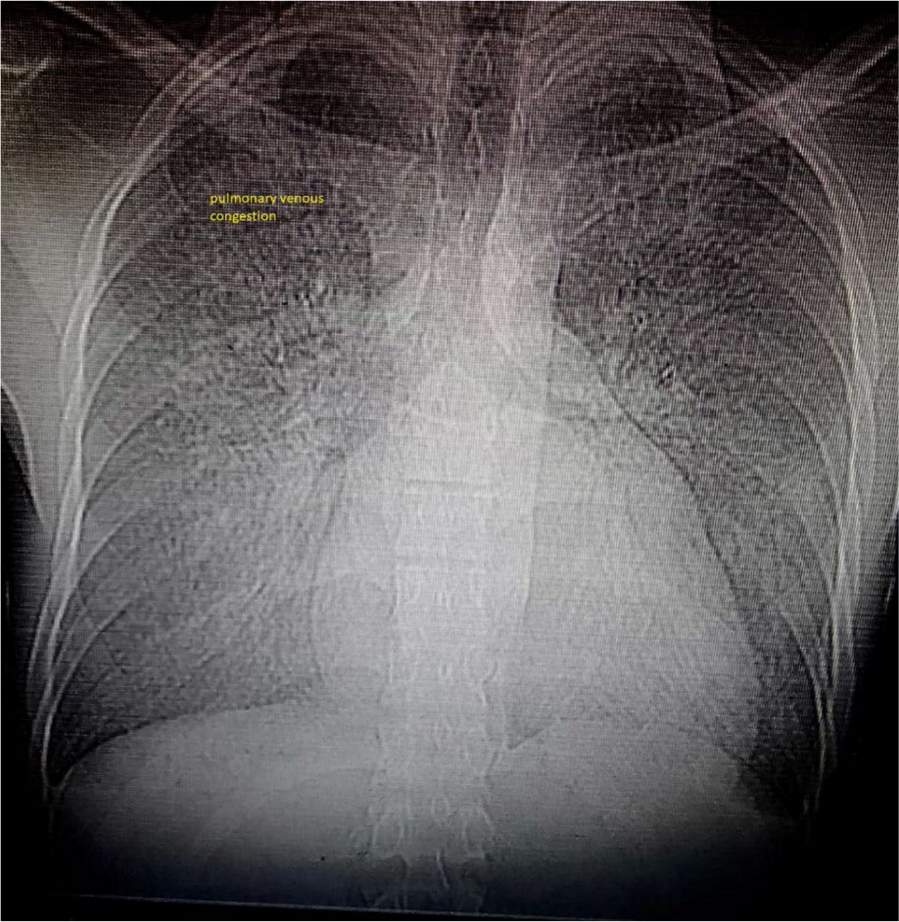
Chest X-ray showed increased cardiothoracic ratio with pulmonary venous congestion
